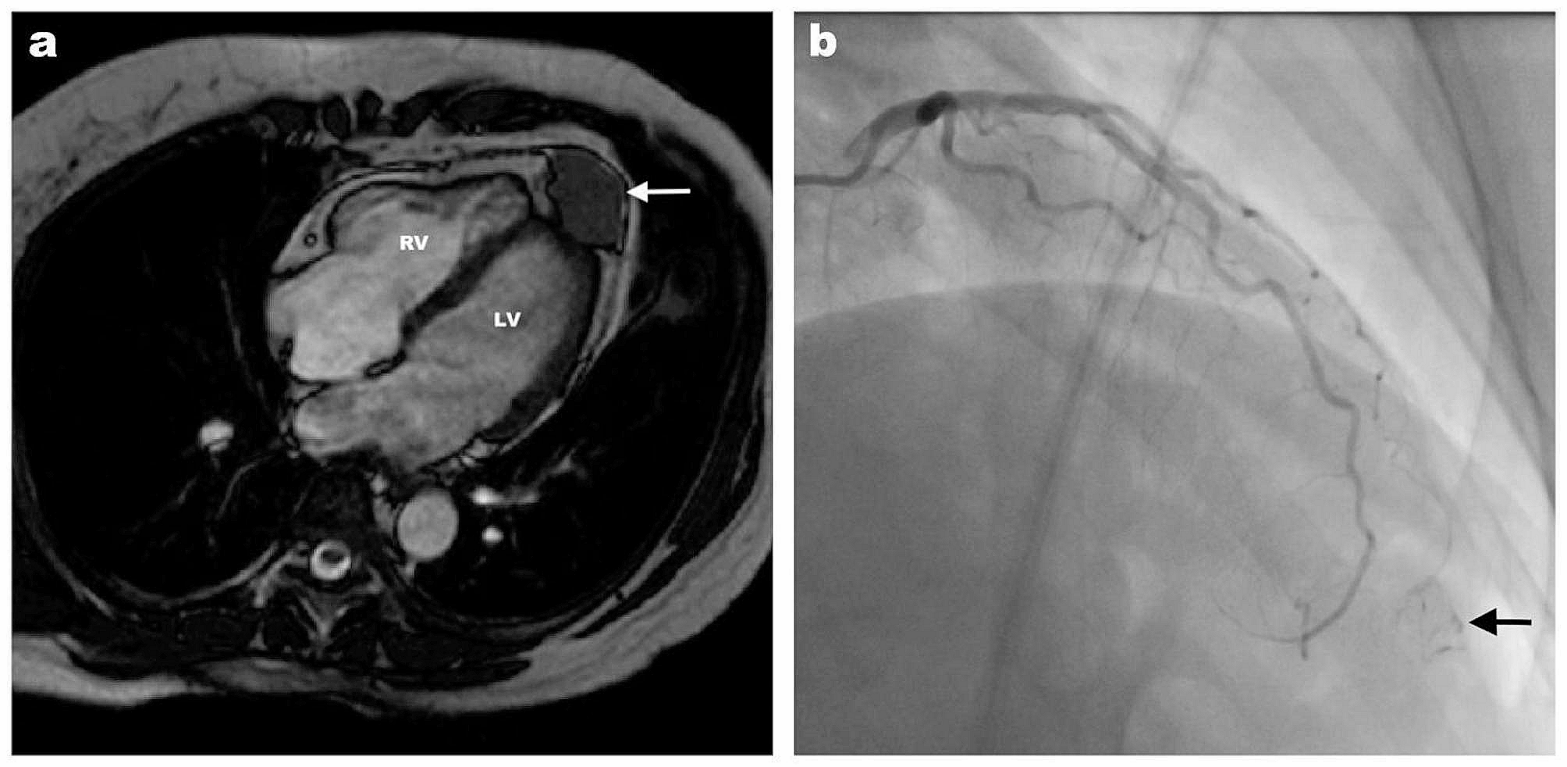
MRI of the suspected cardiac metastasis of the ULMS. a) A cardiac MRI shows an intrapericardial tumour (white arrow) adjacent to the apex of the left ventricle. LV—left ventricle; RV—right ventricle. b) Coronary artery angiography shows tumour vascular supply from the left anterior descending artery (black arrow)
